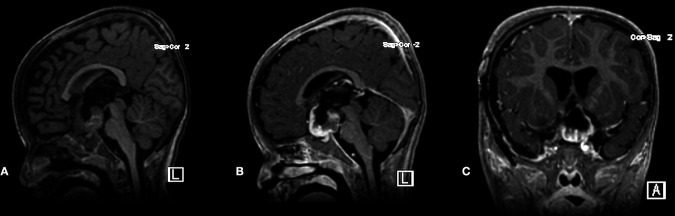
Postoperative plain sagittal T1-WI MRI scan and post-contrast T1-WI sagittal and coronal MRI scans demonstrated adequate decompression and stable residual tumor at 6-month follow-up.
